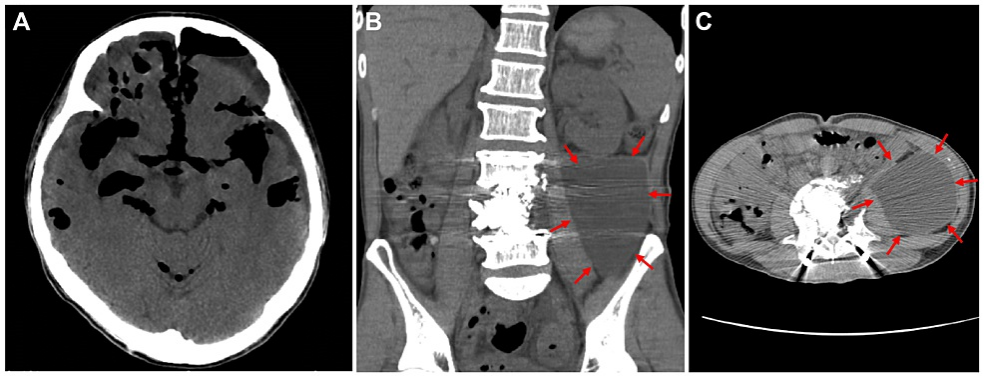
A CT scan shows a large amount of air in the cranium (A) and massive fluid retention in the abdomen (arrows, B: coronal and C: axial).
